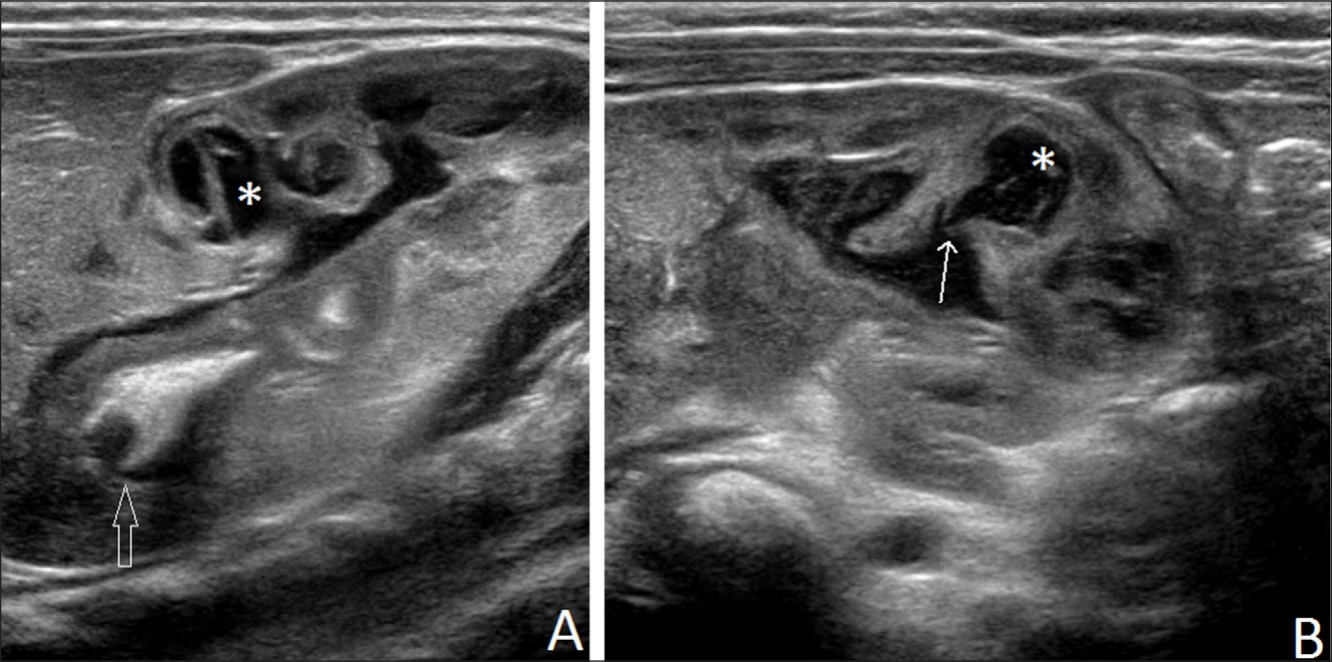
(A) Longitudinal ultrasound scan of the right kidney shows abscesses (asterisk), where at some locations echoic trident-shaped fungus balls (open arrow) are seen. (B) There are communications (arrow) between abscesses and dilated pelvi-caliceal cavity (asterisk).
